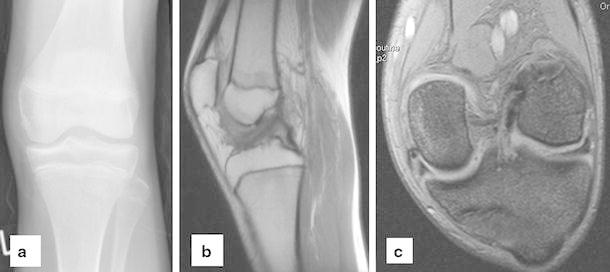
Anteroposterior radiograph of the left knee (a), sagittal MRI (b), and coronal MRI (c) at 24-month follow-up with intact ACL and without detectable disturbance of the growth plate of the distal femur
